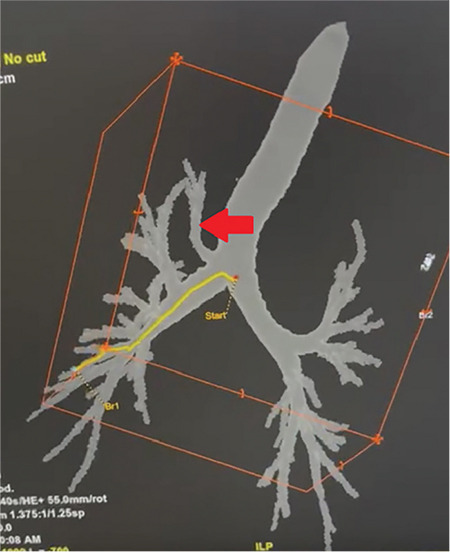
Computed tomography image of our patient's tracheal bronchus. The tracheal bronchus (red arrow) is located as the right apical posterior segment.
